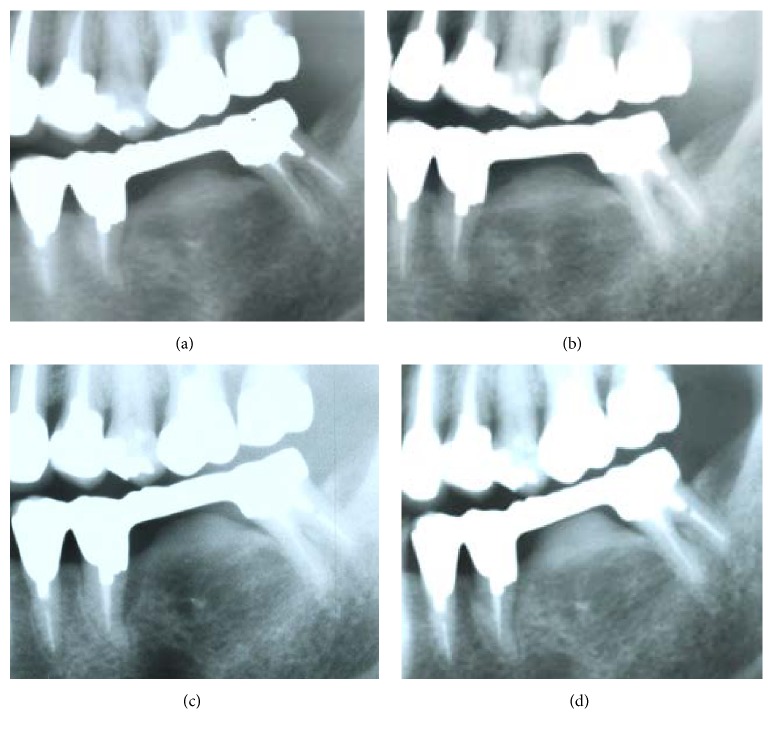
Panoramic radiographic images. (a) Image taken in October 2002 (before starting the oral bisphosphonate [BP]). (b) Image taken in February 2005 (before starting the oral BP). (c) Image taken in September 2008 (1 year and 4 months after starting the oral BP). (d) Image taken in September 2011 (5 years and 4 months after starting the oral BP). These images show chronological changes in the subpontic bone formation.
